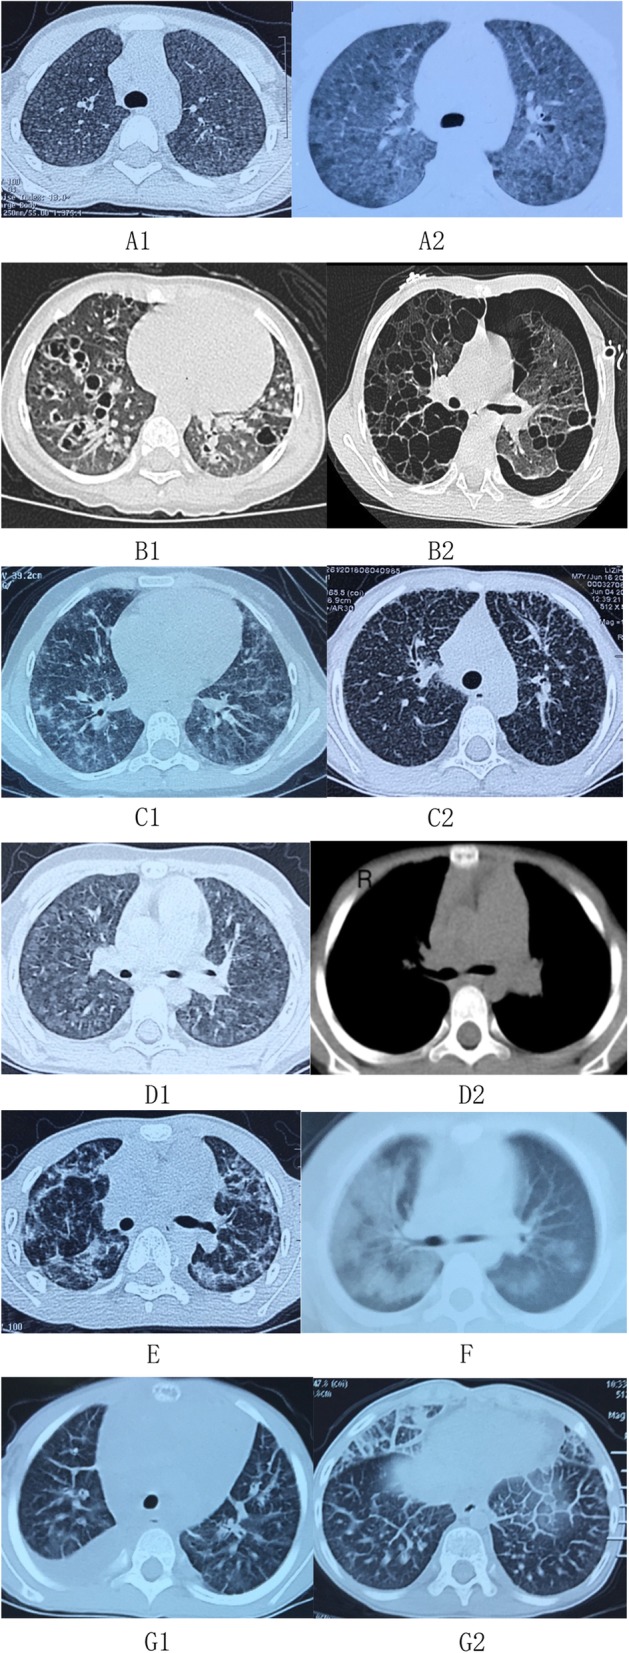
Characteristic HRCT of chILD in children older than 2 years of age. A1 HRCT of hypersensitivity pneumonitis in a patient showing diffuse bilateral small poorly-defined centrilobular nodules. A2 HRCT of hypersensitivity pneumonitis in another patient showing diffuse bilateral ground-glass opacities with areas of air trapping. B1 HRCT of langerhans cell histiocytosis (LCH) in a patient showing bilateral cysts and nodules. B2 HRCT of LCH in another patient showing bilateral cysts and pneumothorax. C1 HRCT of common variable immunodeficiency (CVID) with granulomatous-lymphocytic interstitial lung disease (GLILD) in a patient showing reticulonodular opacities with bilateral ground-glass macronodular opacities. C2 HRCT of COPA syndrome in a patient showing a lymphocytic intestinal pneumonia (LIP) pattern characterized by bilateral diffuse reticulonodular opacities. D1 and D2 HRCT of methylmalonic acidemia (MMA) and homocysteinemia in a patient showing diffuse bilateral poorly defined ground-glass nodules and enlarged pulmonary artery. E HRCT of cryptogenic organizing pneumonia (COP) in a patient showing bilateral liner opacities and consolidations in peripheral distribution. F HRCT of diffuse alveolar hemorrhage (DAH) in a patient showing bilateral diffuse bilateral ground-glass opacities. G1 and G2 HRCT of a patient with diffuse pulmonary lymphangiomatosis (DPL) showing diffuse bilateral grossly thickened interlobular septal and pleural effusion
